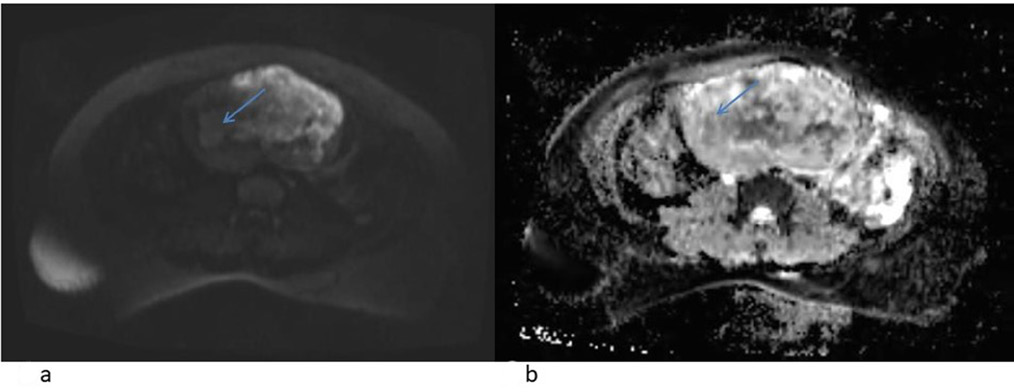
(a, b): DWI with ADC images showing mild diffusion restricting
